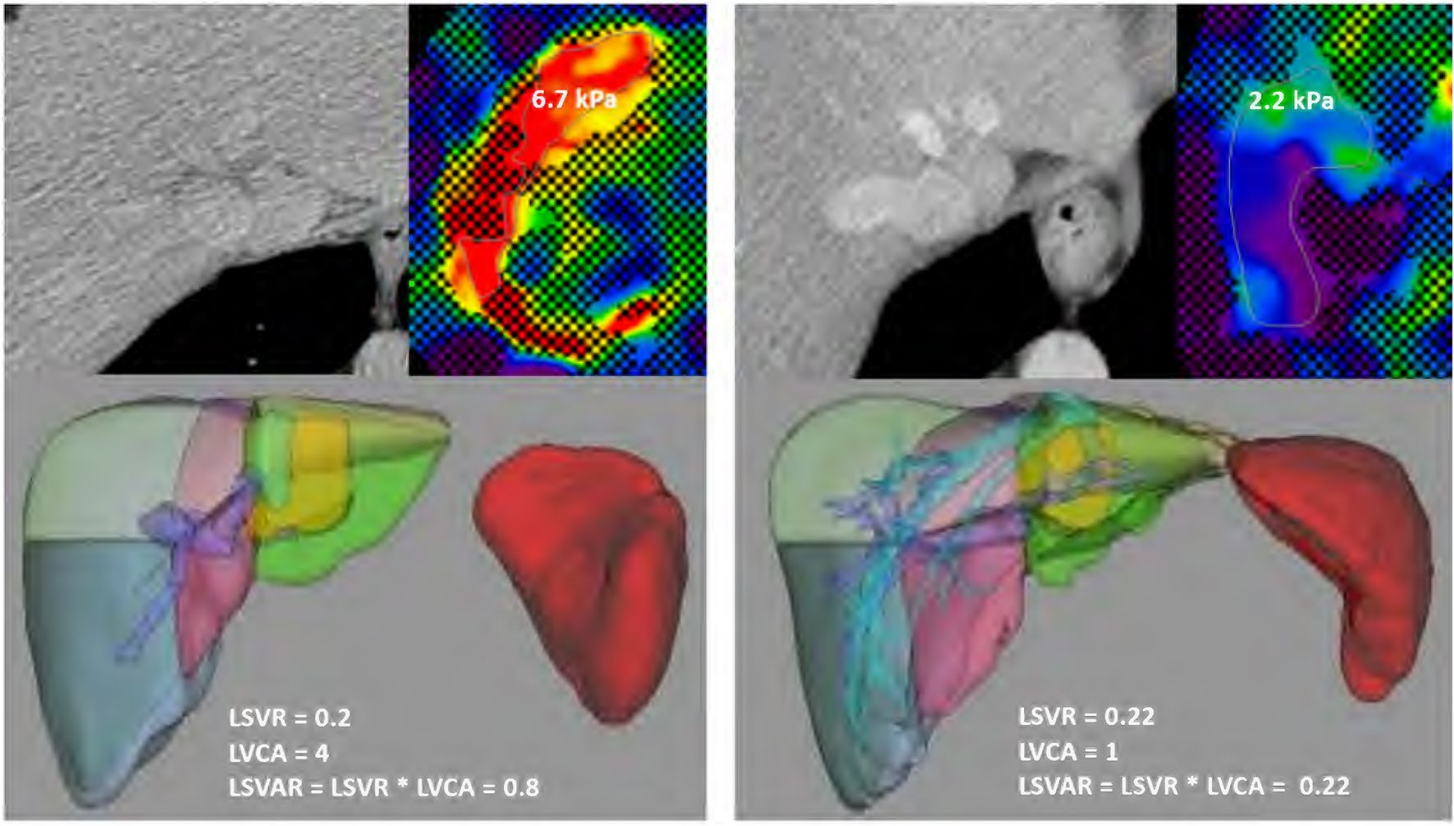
Patient examples with and without clinically significant increased liver stiffness demonstrating the LSVR and LSVAR. On the left side, CT images from a cirrhotic patient (liver stiffness of 6.7 kPa) with hypertrophic left and caudate lobes as well as noncontrasted liver veins (LVCA 4) are shown, resulting in an LSVR = 0.2 and LSVAR = 0.8. On the right side, CT images from a patient with normal liver stiffness are shown (2.2 kPa). All liver fibrosis measures were within normal limits; LSVR = 0.22, LSVAR = 0.22 and LVCA = 1. *LVCA* liver vein to cava attenuation, *LSVR* liver segmental volume ratio, *LSVAR* liver segmental volume and attenuation ratio
